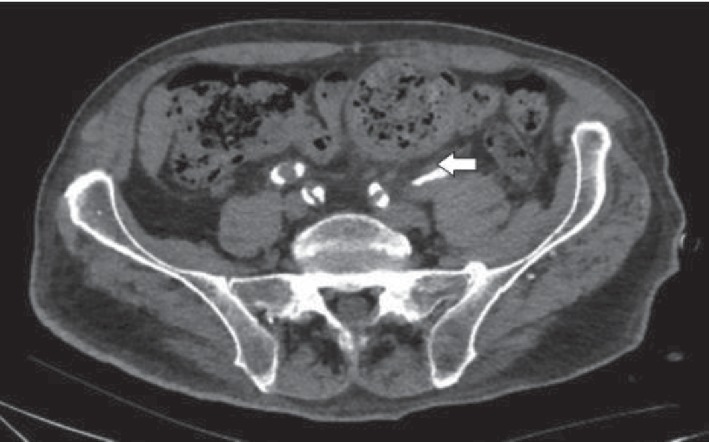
Follow-up noninjected computerized tomographic (CT) scan of the abdomen and pelvis. Resolution of the fecaloma and patency of the left ureter (arrow).
